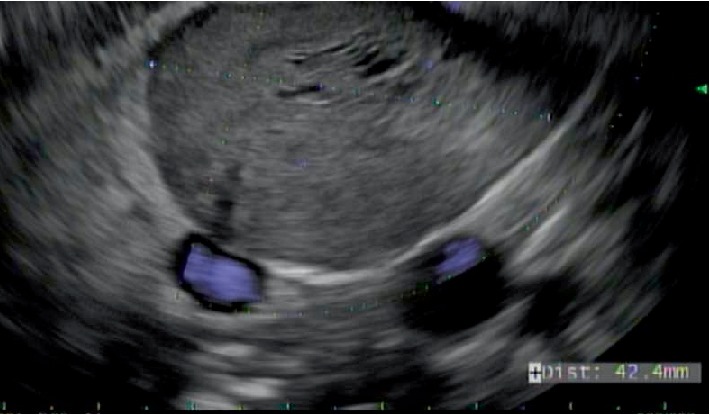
Endoscopic Ultrasound (EUS) examination demonstrated an oval, hypoechoic mass with well-defined borders and no evidence of associated regional lymphadenopathy. No invasion of regional vasculature was present.
